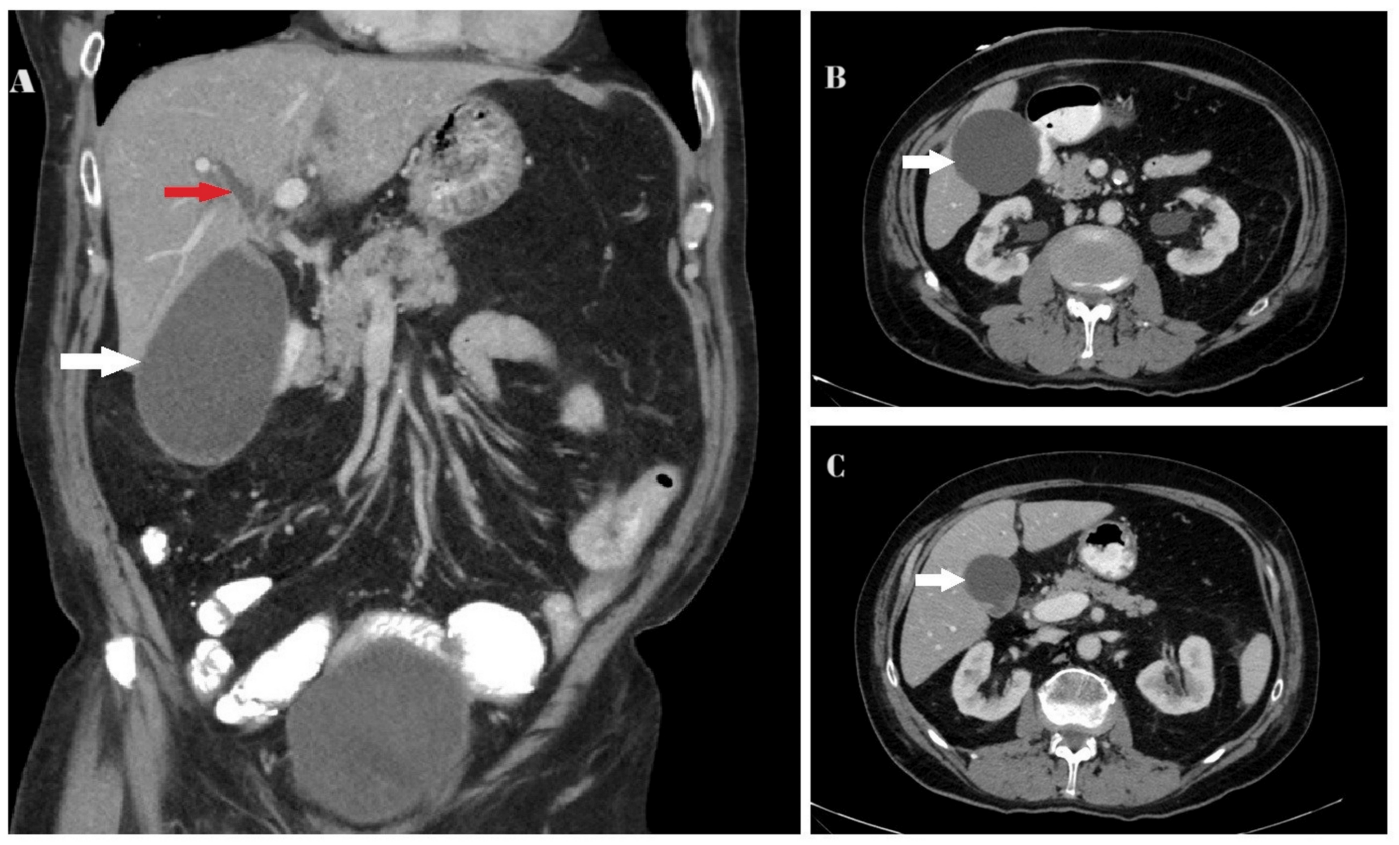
CT scan images of the abdomen and pelvis (A) Coronal view showing marked gallbladder distention (white arrow) and early intrahepatic biliary dilatation (red arrow). (B) Axial view, white arrows indicating gallbladder distension at the level of T12. (C) Axial view, white arrows indicating gallbladder distension at the level of L2 CT, computed tomography; L2, lumbar vertebral 2; T12, thoracic vertebral 12
